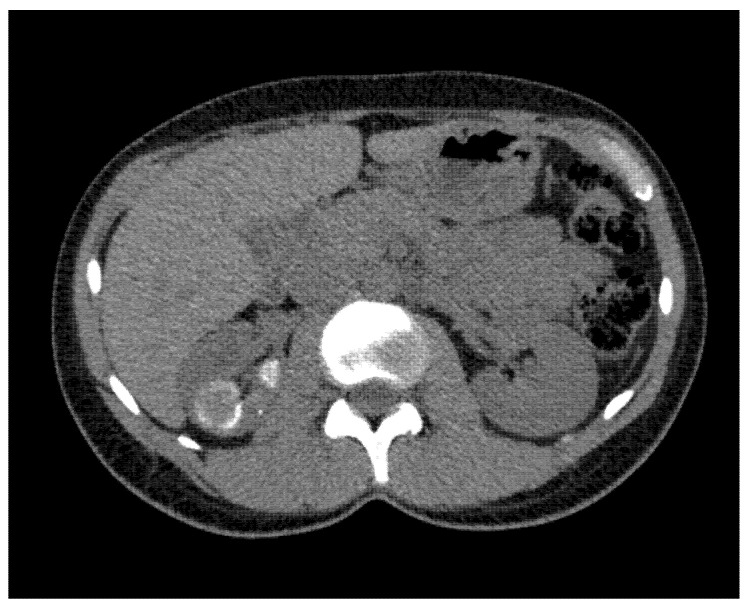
CT Scan image showing right upper pole kidney TB lesions—transversal view.
